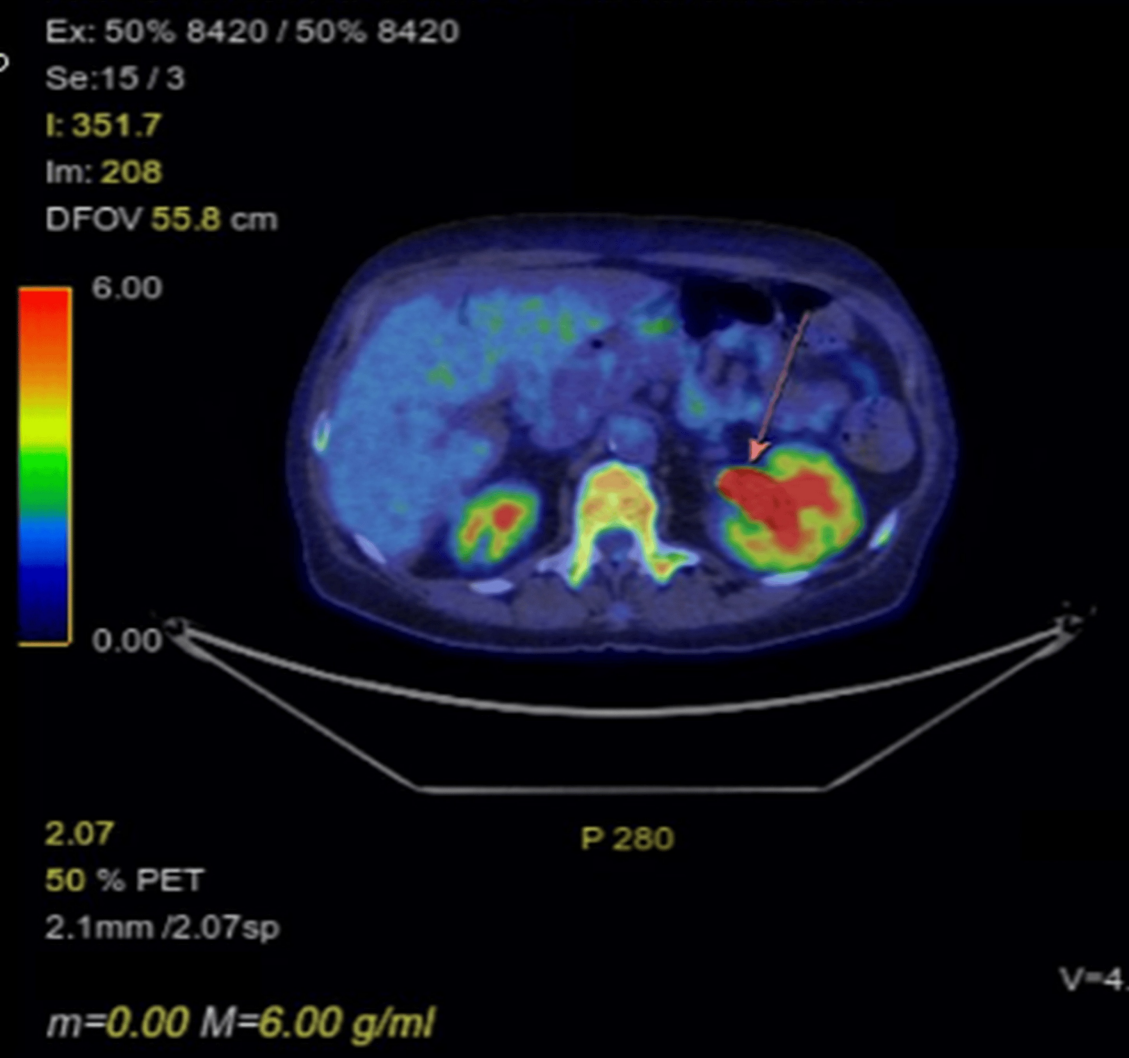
PET-CT showing left hydroureteronephrosis (arrow)
